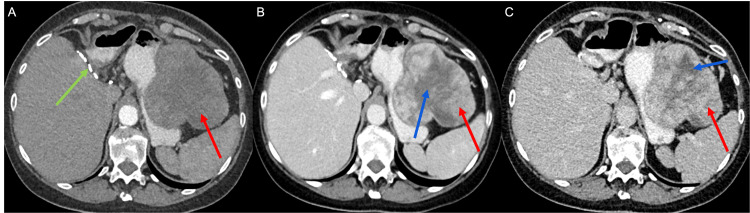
Axial CT images of the abdomen without contrast (A) during the arterial phase (B) and during the portovenous phase (C) demonstrate an exophytic heterogeneous mass involving the greater curvature of the stomach (red arrows in A, B, and C). There is effacement of the gastric lumen, similar to the imaging a month prior. The mass enhances on the arterial phase and has internal streaky hypodensities on the arterial (blue arrow in B) and portovenous (blue arrow in C) phases. No calcifications are present as noted by the non-contrast image; surgical staples from prior left hepatectomy are present (green arrow in A).
